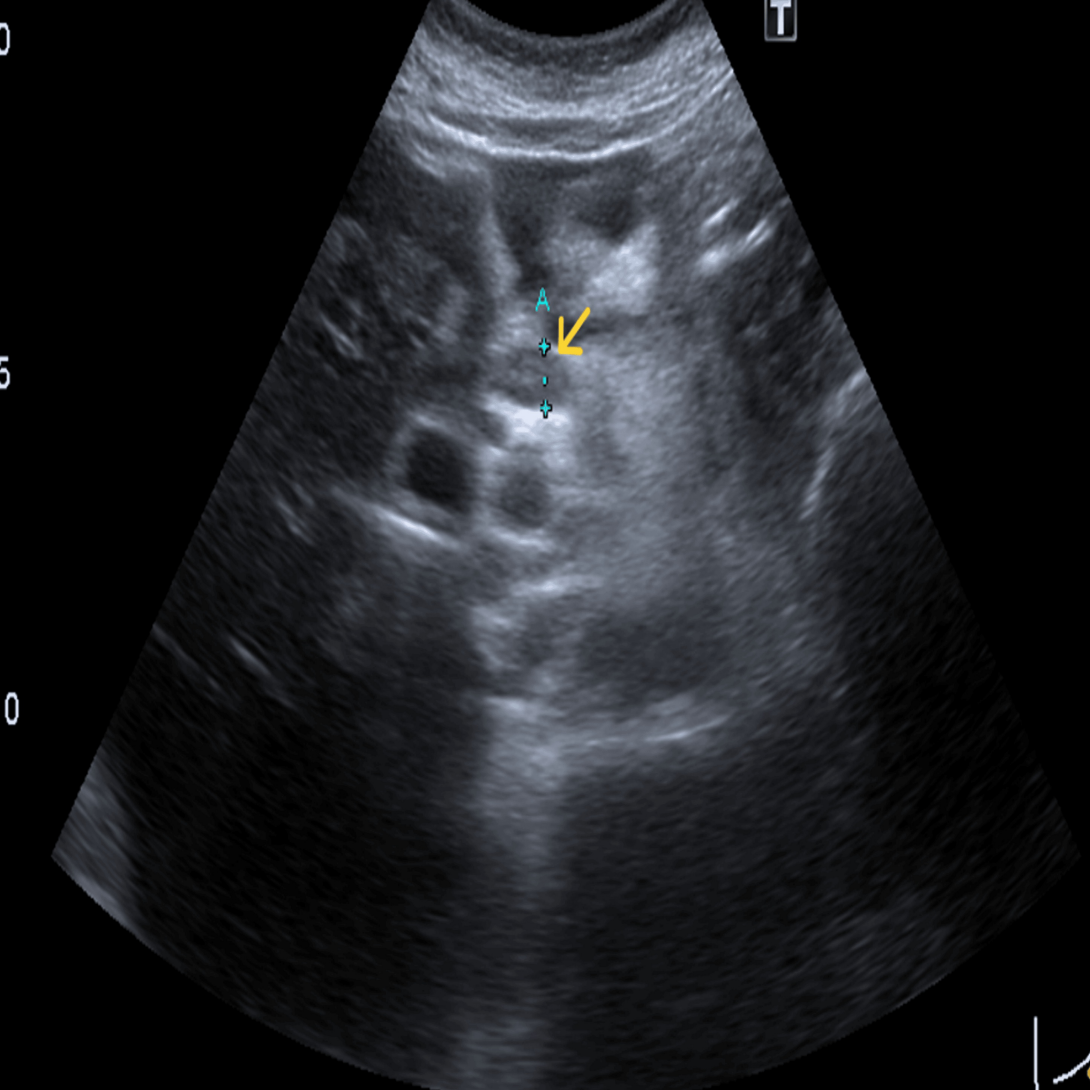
Abdominal ultrasound of hepatic ganglia at the level of the hepatic hilum
